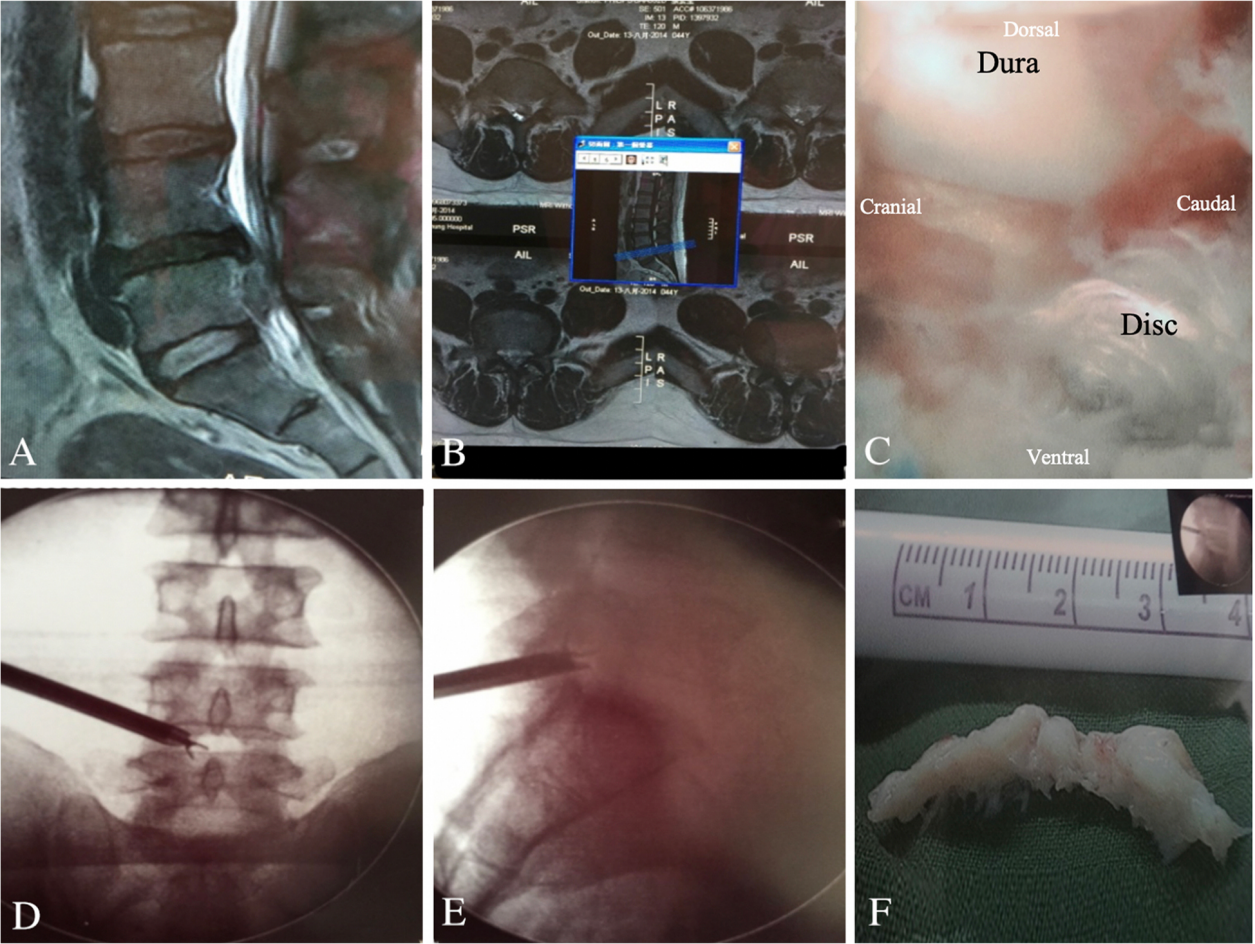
L4–L5 TELD changed to L5–S1 IELD for L4–L5 high-grade downward migration. a Preoperative MRI lateral view showing high-grade downward migrated disc herniation at the L4–L5 level. b Preoperative MRI axial views showing disc herniation at the L4–L5 level. c Intraoperative fluoroscopic view of the herniated disc material. d Fluoroscopy anteroposterior view showing forceps near the disc fragment during L4–L5 TELD. e Fluoroscopy lateral view showing the forceps grasping the disc fragment during L5–S1 IELD. f Removed disc fragments
